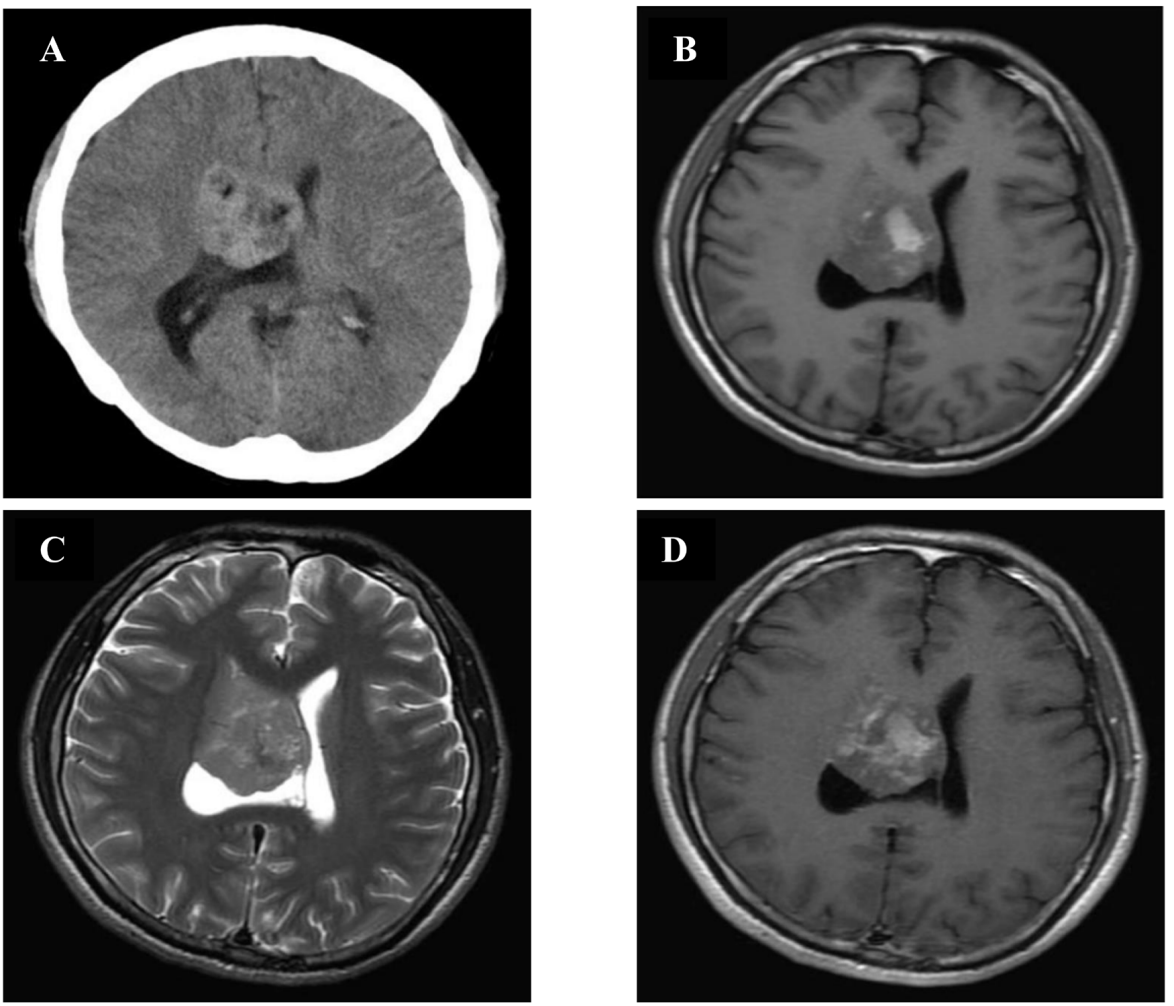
CT and MRI of patient 1 A. CT showed a solid lesion in the right lateral ventricle, with fat component (hypodense). (B., C., D.) Brain MRI (axial T1WI and T2WI, gadolinium-enhanced T1WI) showed an irregular mass measuring 4.5cm in diameter with a definite boundary in the right lateral ventricle. The mass had heterogeneous components: neuronal (hypo- to isointensity on T1WI and isointensity on T2WI) and fat (strongly T1WI hyperintensity and moderately T2WI hyperintensity). Contrast enhancement of the mass was slight and inhomogeneous.
